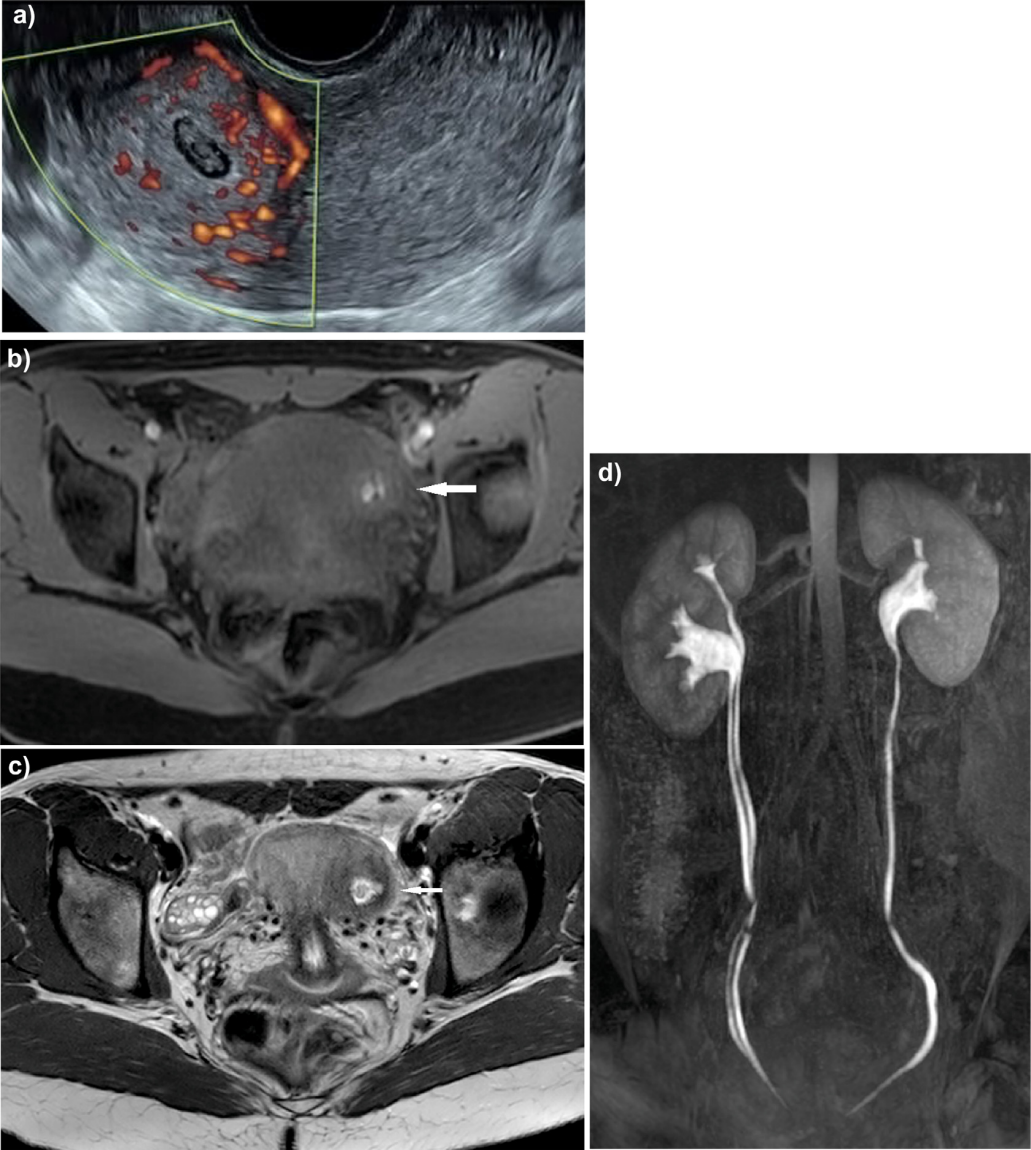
17-year-old nulliparous girl with intense dysmenorrhea since the age of 16 years. a: Transvaginal ultrasound with Doppler revealed in heterogenous hypoechoic meaning the presence of blood clots in the left part of the myometrium. It was surrounded by vascularization. b: T2-weighted sequence - axial view. The lesion was located in the left part of the uterus near the origin of the round ligament (↑white arrow). The heterogenous inner part indicated the presence of blood clots. The ovaries were normal (only the right one is visible in this slice). c: T1-weighted sequence – axial view. The hypersignal inside the inner part of the llesion indicated the presence of blood (↑white arrow). d: MR Urography-coronal view. A minor congenital urinary tract abnormality was present: duplication of the right urinary system. (Color version of the figure is available online.)
